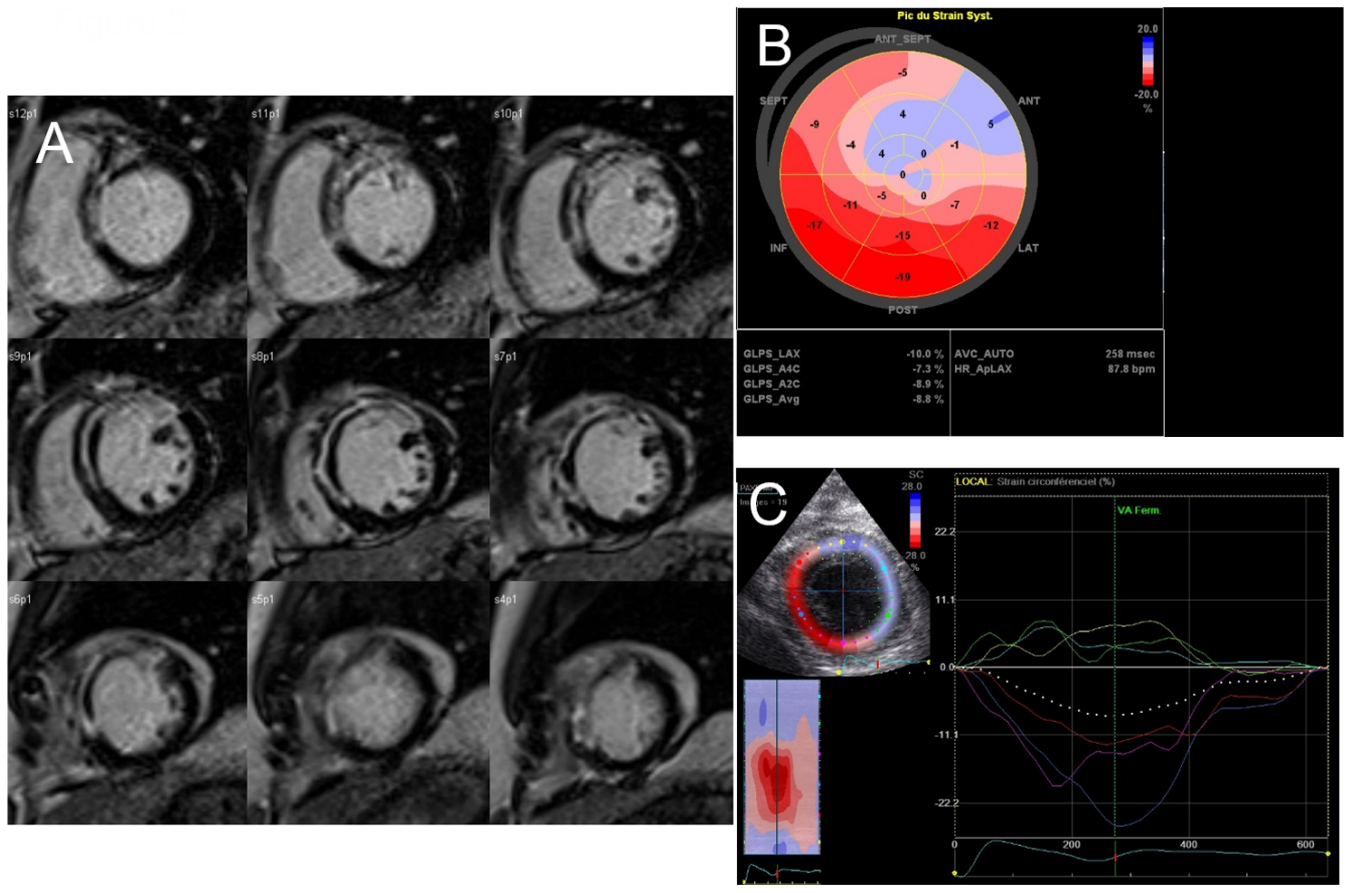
A case of anterior infarction related to the occlusion of the proximal left anterior descending coronary artery. A, CMR late gadolinium hyperenhancement slices of left ventricular short axis showing transmural extension of myocardial infarct in anterior and antero-septal zones and central hypoenhancement defining microvascular obstruction; B, longitudinal global strain represented on bull’s eye view; there is a severe dyskinesia (blue color) of the anterior wall; C, dyskinesia is also observed in parasternal view when considering the circumferential deformation of this severe transmural infarction.
